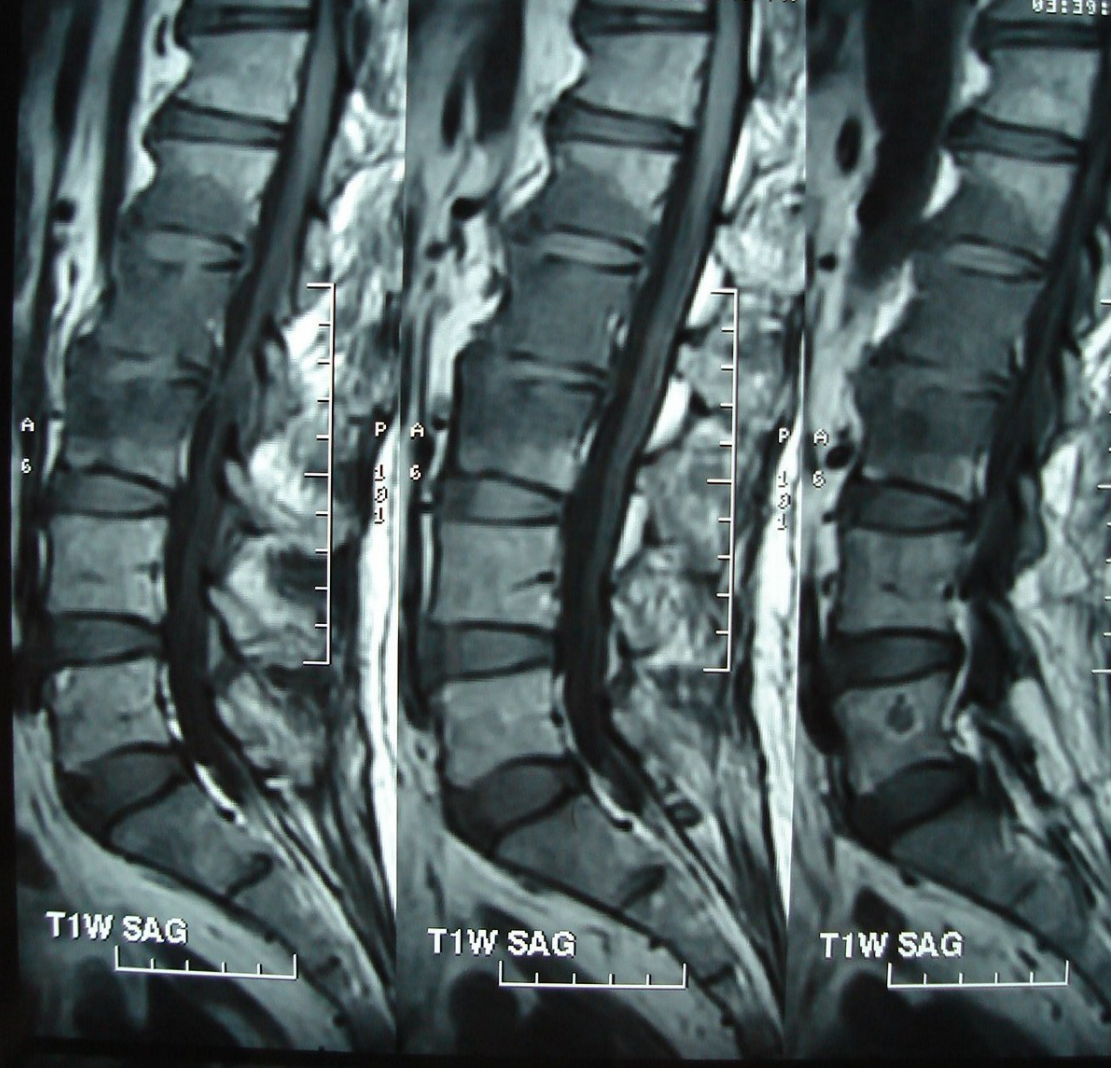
sagittal T2 MRI showing diskitis and vertebral signal changes suggestive of osteomyelitis
